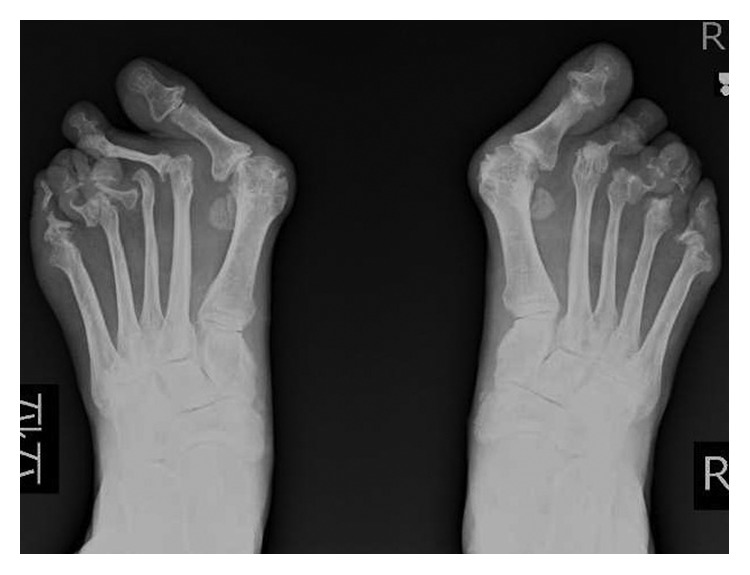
Plain radiographs of the feet.
